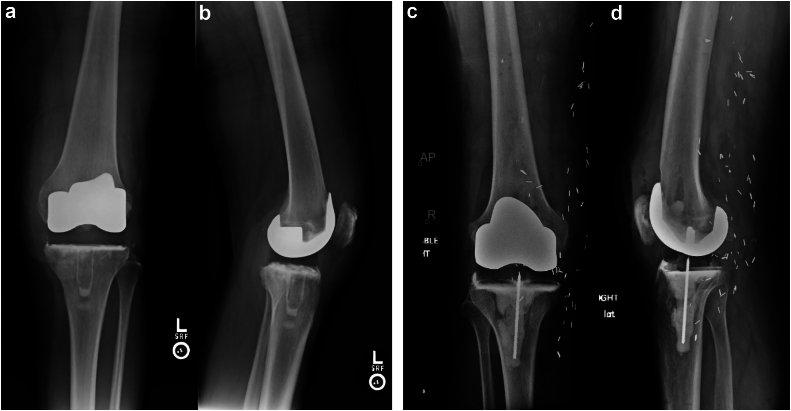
(a and b) Postoperative X-ray showing left all-polyethylene tibia (APT) at 6 weeks. (c and d) Postoperative X-ray showing right polyethylene insert (PI) at 6 weeks.
